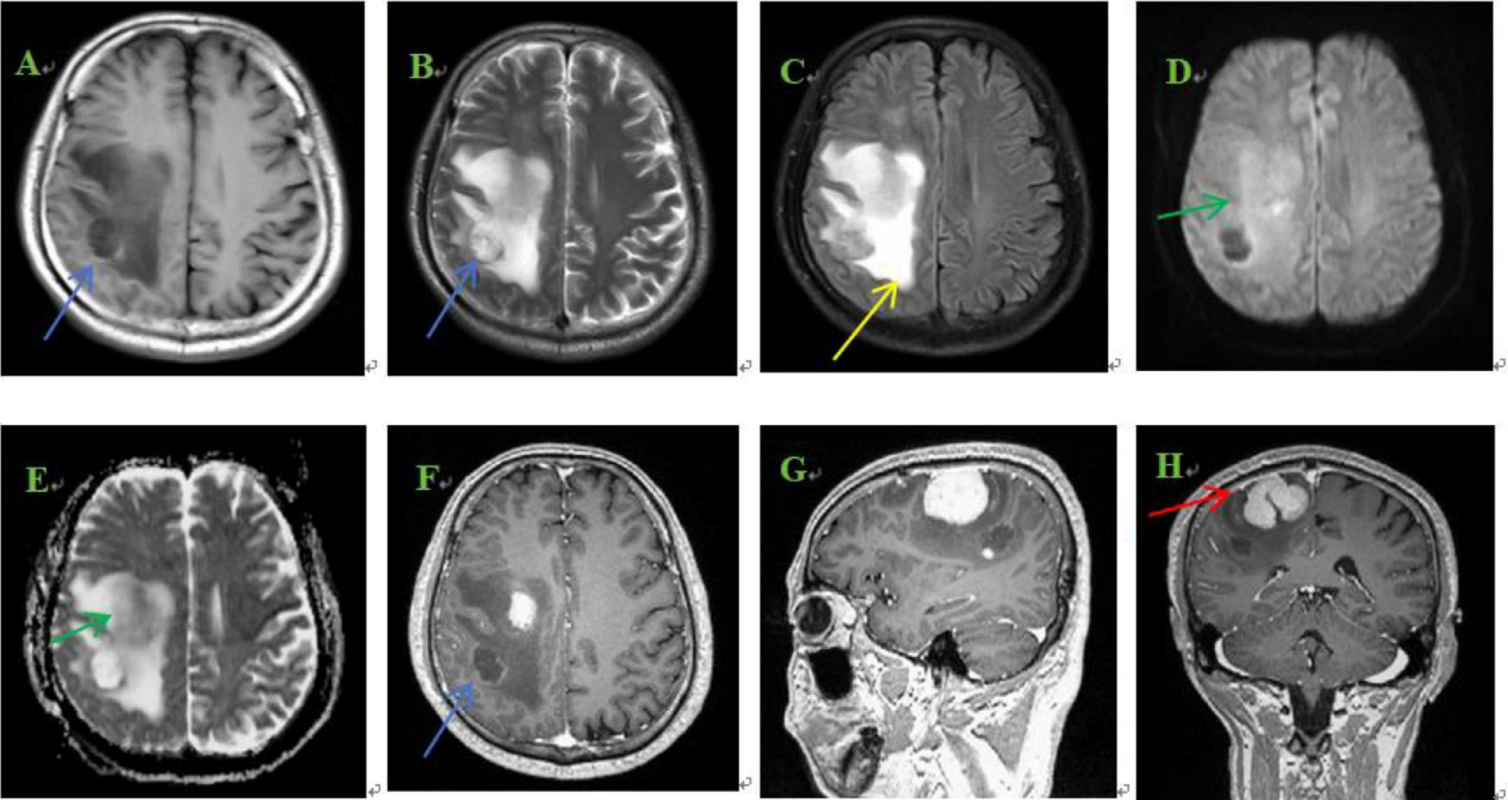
MRI images. (A-E) Axial T1WI, T2WI, T2-FLAIR, DWI, and ADC images, respectively; (F-H) Axial and sagittal contrast-enhanced scans. The images reveal nodular and mass-like lesions in the right fronto-parietal lobe, displaying hypointense T1 and slightly hyperintense T2 signals, with extensive peritumoral edema (yellow arrow). Contrast-enhanced images show significant enhancement, with cystic and necrotic areas visible within the lesion (blue arrow). There is a clear mass effect and infiltration of the adjacent dura mater (red arrow). On DWI, part of the lesion exhibits mild diffusion restriction (green arrow).
